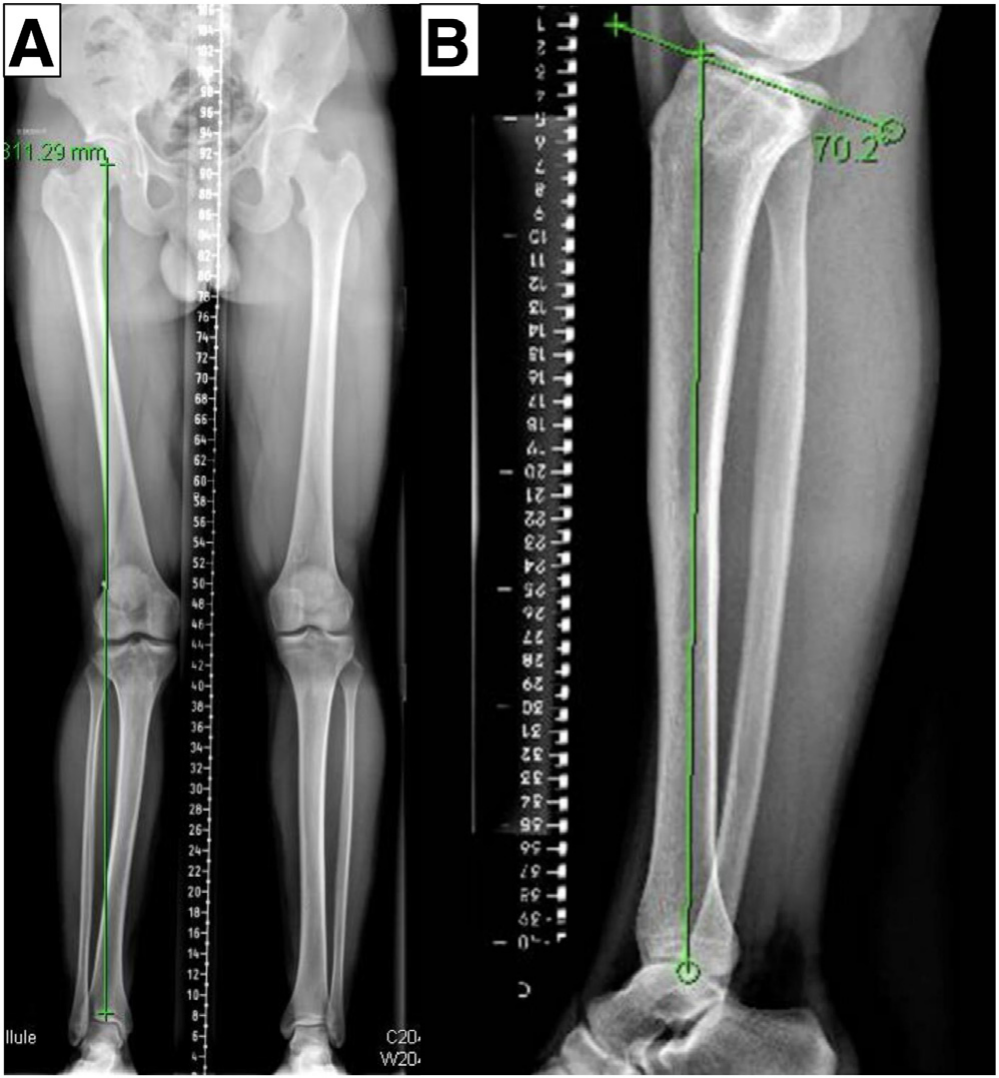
Preoperative long-leg radiographs (right knee). (A) Marked valgus deformity is observed in the right knee. (B) Posterior tibial slope is approximately 20°.
